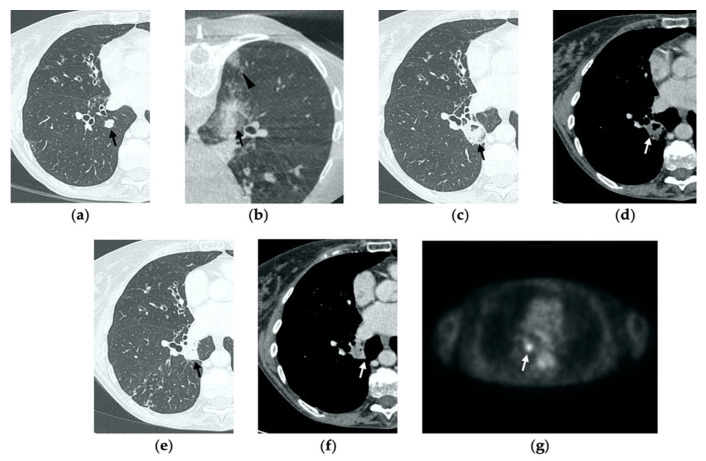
77-year-old-woman with pulmonary metastasis from CRC in the right lower lobe. (a) Axial CT images prior to treatment (black arrow). (b) Prone cone-beam CT image post-MWA shows GGO of the ablation site (black arrow) and alveolar hemorrhage along the needle tract (arrowhead). (c,d) Axial 1-month follow-up CT shows a subpleural consolidation with well-defined margins, inner hypoattenuating bubbles, a central cavitation (black arrow) and no contrast enhancement (white arrow). (e,f) Axial 6-month follow-up CT images demonstrate resolution of the cavitation, decrease in size of the consolidation (black arrow) and focal uptake of contrast material (white arrow) consistent with a diagnosis of residual disease. (g) The diagnosis is confirmed by PET/CT performed at 7 months (white arrow).
